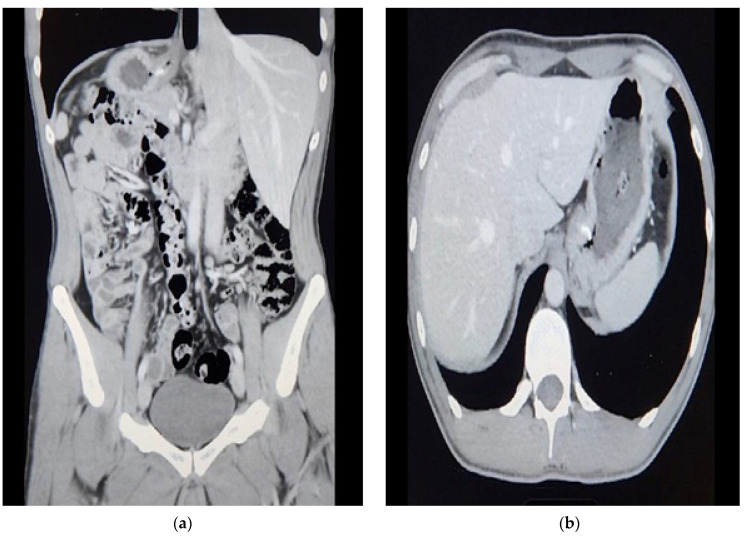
CT scan of the abdomen pelvis: (a) Coronal; (b) Transverse. Suspicious increased wall thickening of the proximal stomach extending to the gastroesophageal junction.
